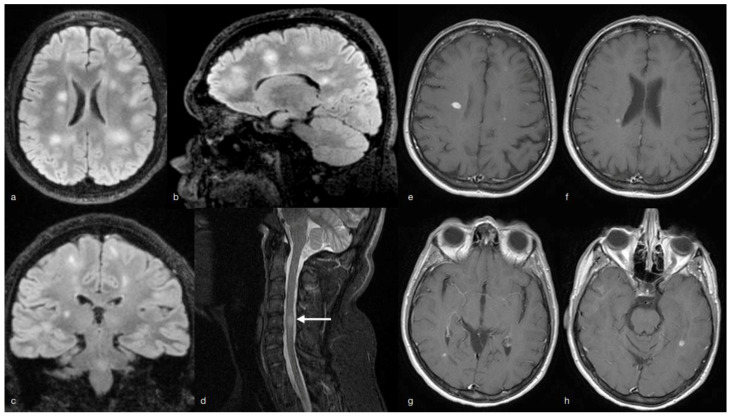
Multiple subcortical, perivenular and periventricular hyperintense lesions in FLAIR (a–c). A paramedian intramedullary with swollen C5 hypertense lesion in STIR (d). Case 2: active lesion with enhancement in T1 gad + (e–h).
